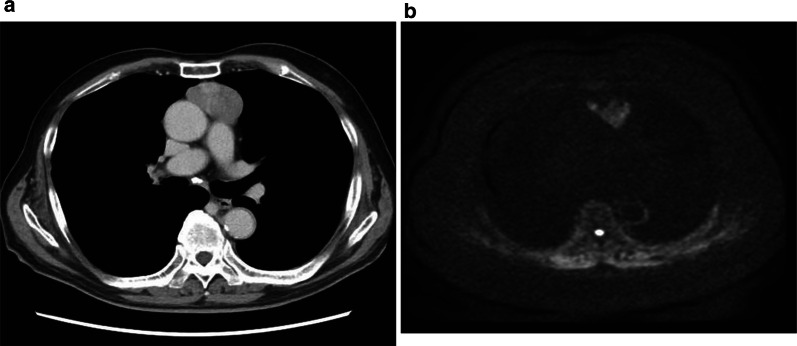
Tumor appearance on diagnostic imaging. a Computed tomography showing a solitary tumor in the anterior mediastinum. b Diffusion-weighted magnetic resonance image showing a high-intensity signal in the tumor
